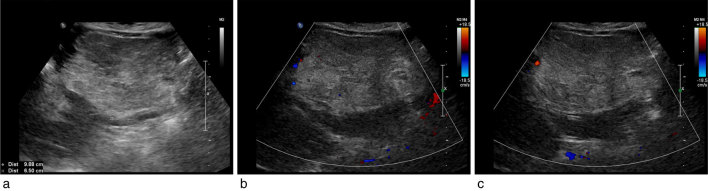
Abdominal ultrasound shows iso-hyperechoic (a) lesion in the left lobe of the liver and its Doppler analysis (b and c) that shows a peripheral pattern of vascularisation.
